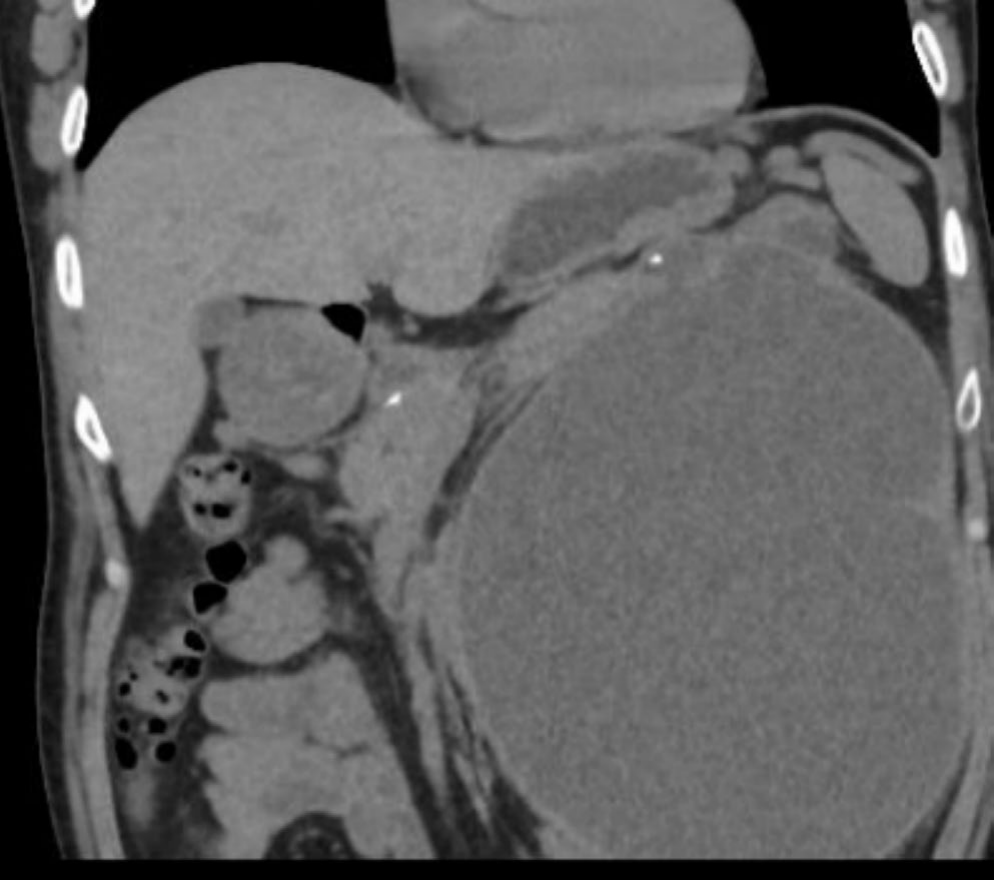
CT examination of the abdomen and pelvis shows pancreatic calcification and a large peripancreatic pseudocyst which was communicating with a dilated pancreatic duct.
